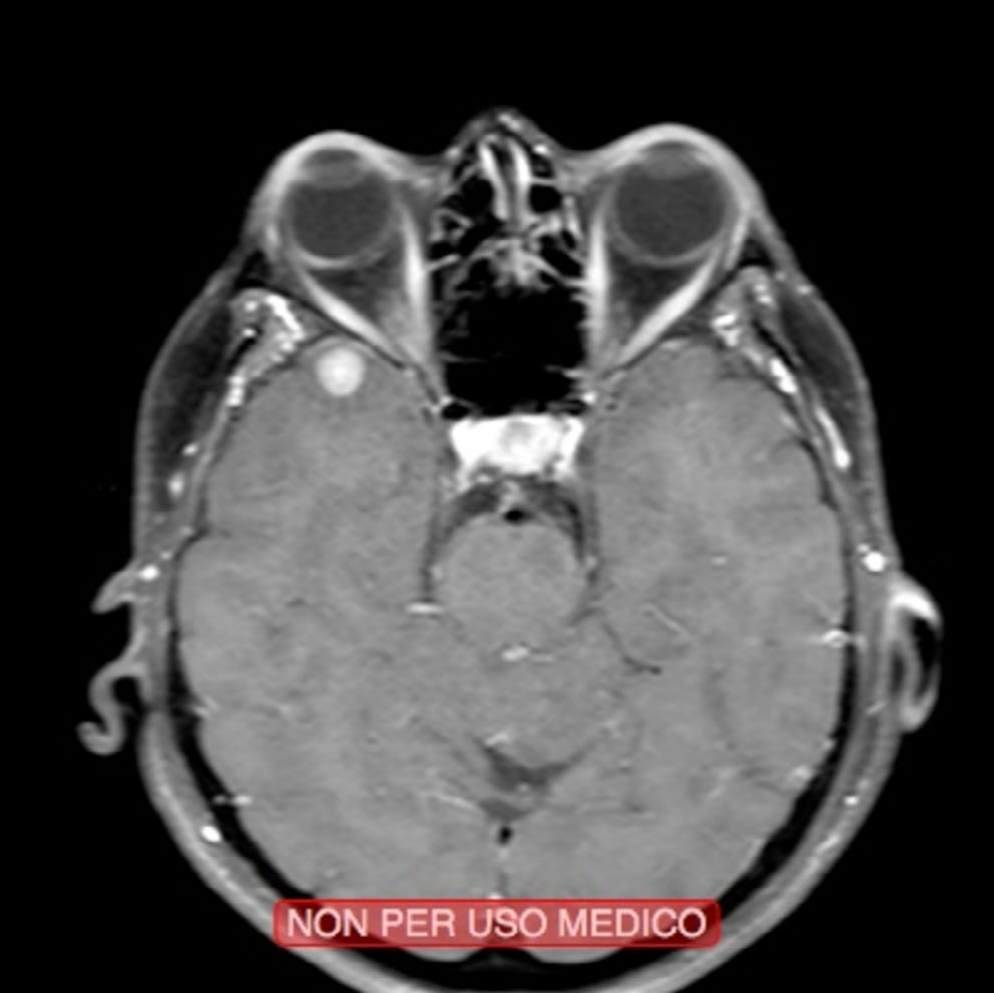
Transversal MRI section showing intracranial lesions, later identified as lung adenocarcinoma metastasis.
